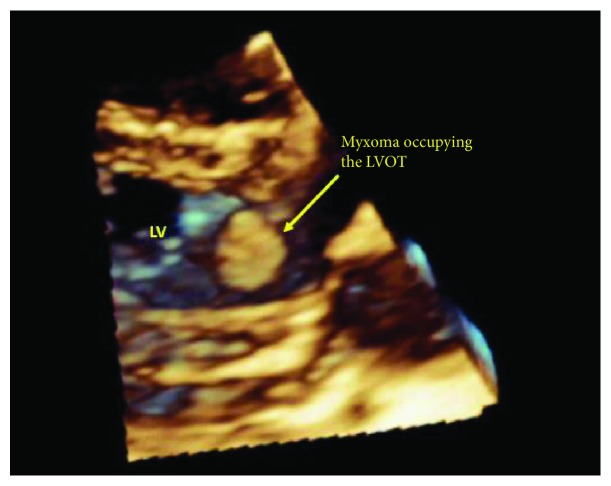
Transthoracic echocardiogram parasternal long-axis view 3-dimensional reconstruction showing a myxoma in the left ventricular outflow tract.
